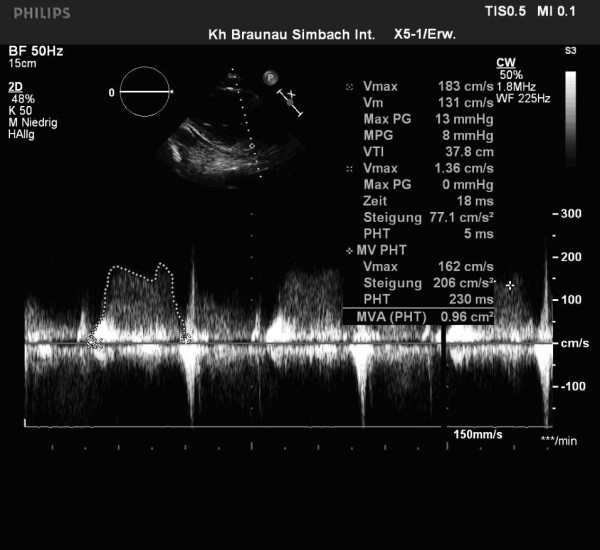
Continous wave (CW) - spectral Doppler tracing indicating mitral stenosis with a mitral valve area less than 1.0 cm2.
